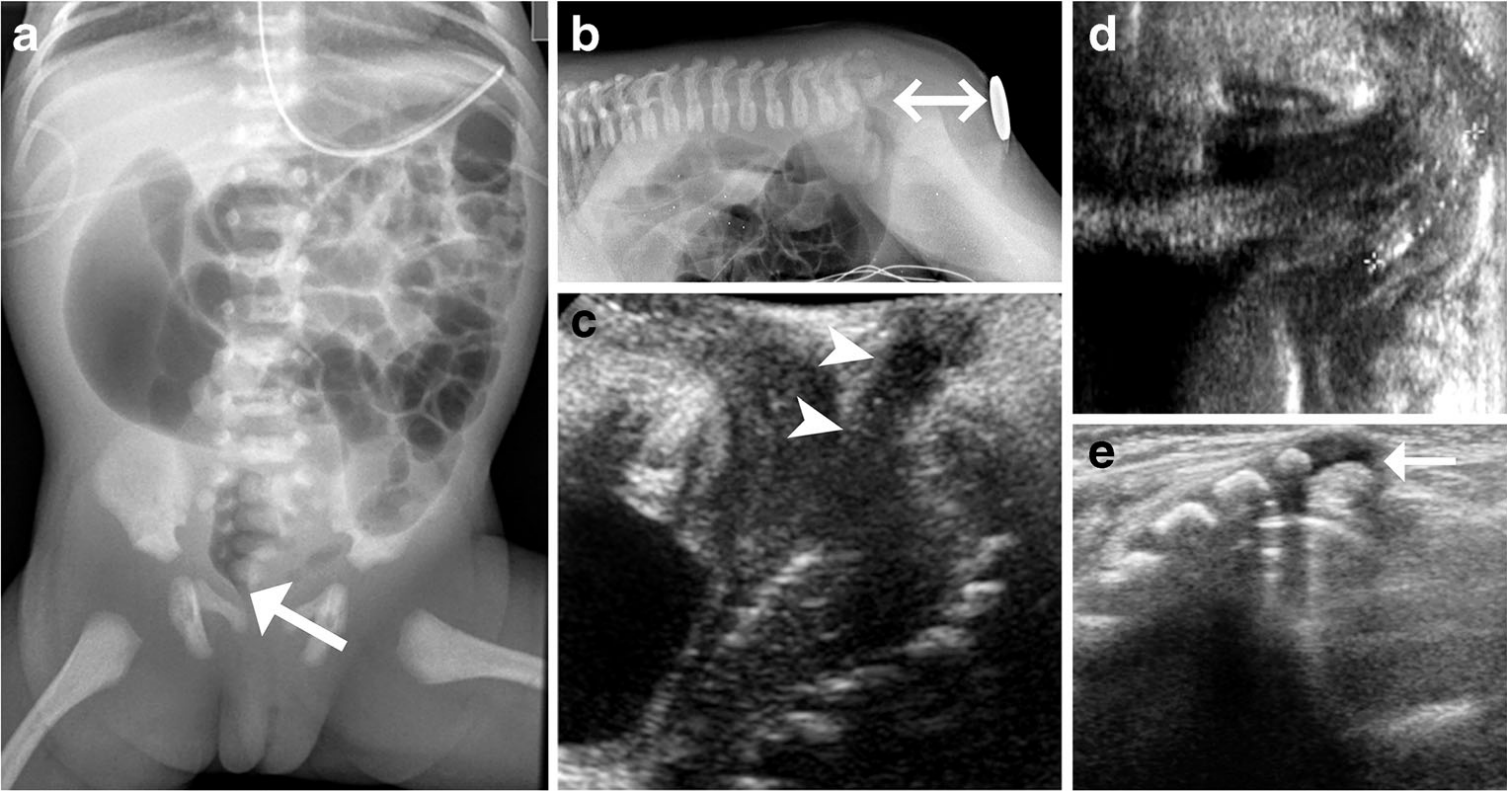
Initial imaging in anorectal and cloacal malformation. In a newborn girl with clinically evident anal atresia, an anterior-posterior abdominal radiograph (a) performed for initial overview and assessment of potentially associated (skeletal) malformations shows minor splaying of the sacral bodies and a high position of the rectal air (*arrow*). Lateral horizontal-beam prone radiograph (b) of the abdomen in a 2-day-old neonate with anal atresia. The area of the external anal opening is indicated by a marker and the distance from the air-filled rectal pouch to the anus (*double arrow*) can be measured if a ruler is added prior to taking the exposure. A newborn boy with anal stenosis: Transperineal US sagittal section (c) demonstrates the normal track of the narrow anal canal (*arrowheads*). Perineal US, sagittal section (d) in a neonate with anal atresia shows an air-filled fistula tract to the perineum (*between calipers*). A supplementary spinal US in prone position (e) with dorsal sagittal intonation shows a hypoplastic cartilaginous coccyx (*arrow*) with an unusually straight course in a 2-day-old girl with cloacal malformation
